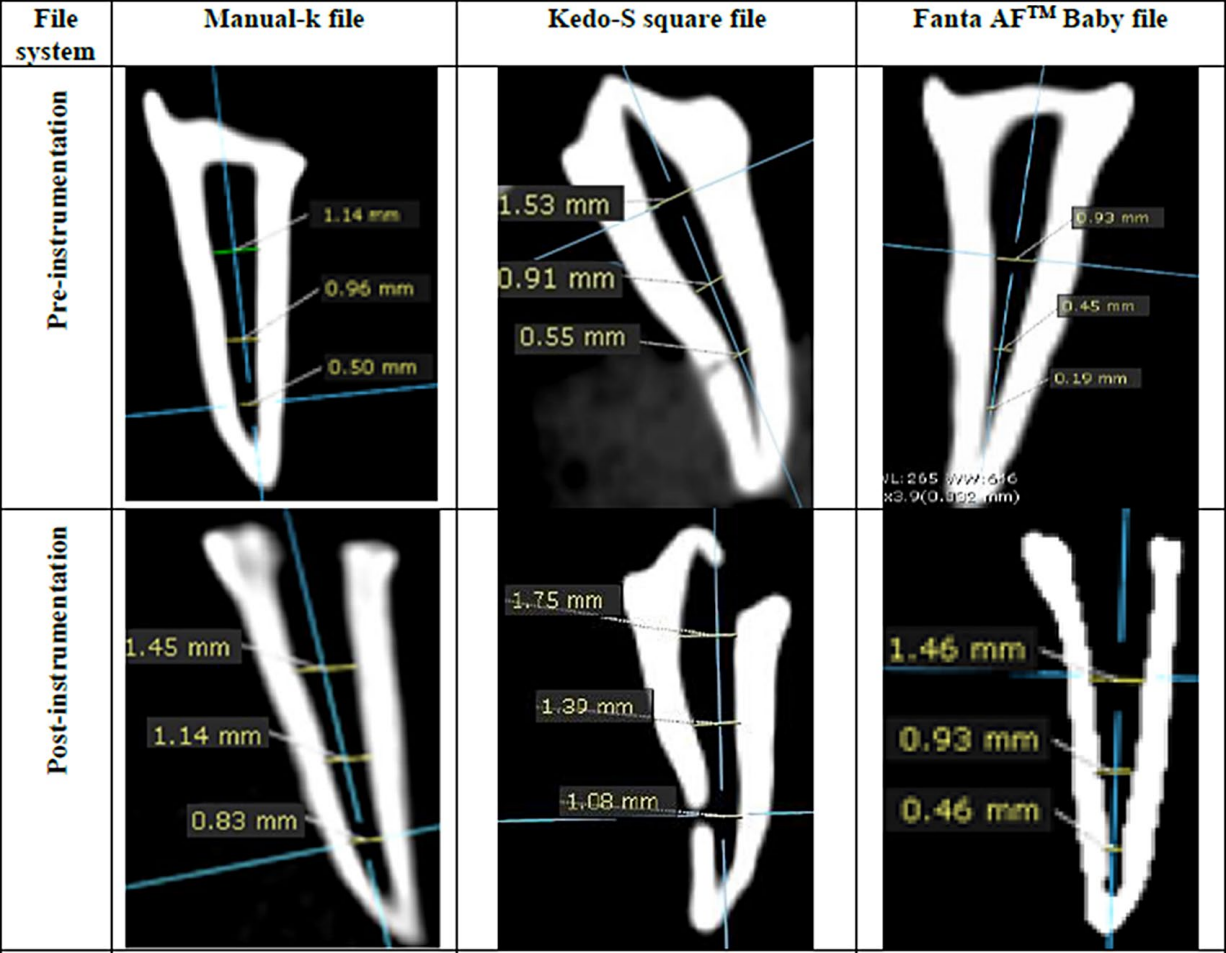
Sagittal cut of CBCT images showing maximum mesiodistal root canal diameter pre- and post-instrumentation with manual K-file, Kedo-S Square file, and Fanta AF^™^ Baby file at cervical, middle, and apical thirds
